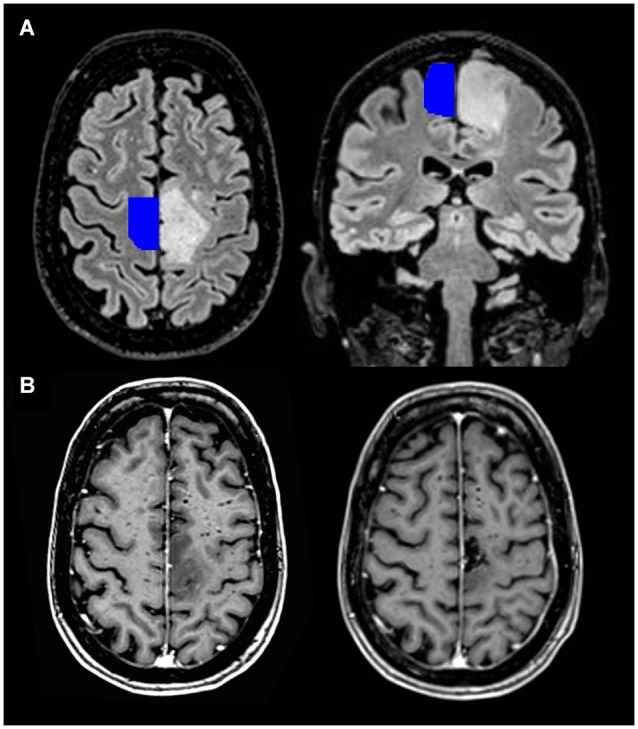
3D view of the probabilistic tractography between both SMA’s from a single healthy subject (made with FSL).^1^ Pre- and postoperative MRI scan of a 64-year-old patient with a diffuse astrocytoma (WHO grade II) in the left SMA. (A) Transversal and coronal T2-weighted FLAIR images, with an SMA template projected on the healthy hemisphere. The latter is freely available and derived from a large meta-analysis describing the location of the sensorimotor areas (Mayka et al., 2006). (B) Transversal images after gadolinium contrast from the same patient before (left lower corner) and three months after the operation (right lower corner). She had a complete motor loss on the right side after the operation, which quickly recovered.
